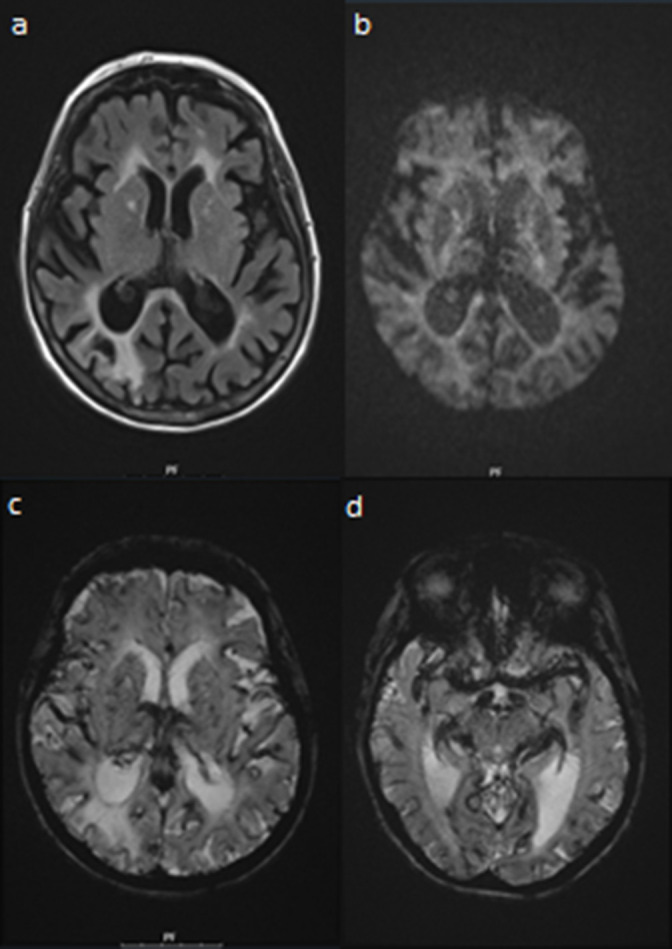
MRI of the brain in a patient 2 days after receiving therapeutic ferumoxytol infusion. (a) FLAIR images demonstrate dark signal at the junction between subarachnoid CSF and the surface of the brain due to intravascular ferumoxytol. (b) Diffusion-weighted images demonstrate no diffusion restriction abnormality and diminished signal in the subarachnoid space due to intravascular ferumoxtyol. (c) Gradient echo imaging demonstrates susceptibility artifact within all visualized intracranial vessels, sparing the parenchyma of the brain due to intravascular ferumoxytol. (d) A more inferior gradient echo image demonstrates similar findings. CSF, cerebrospinal fluid; FLAIR, fluid attenuated inversion recovery.
